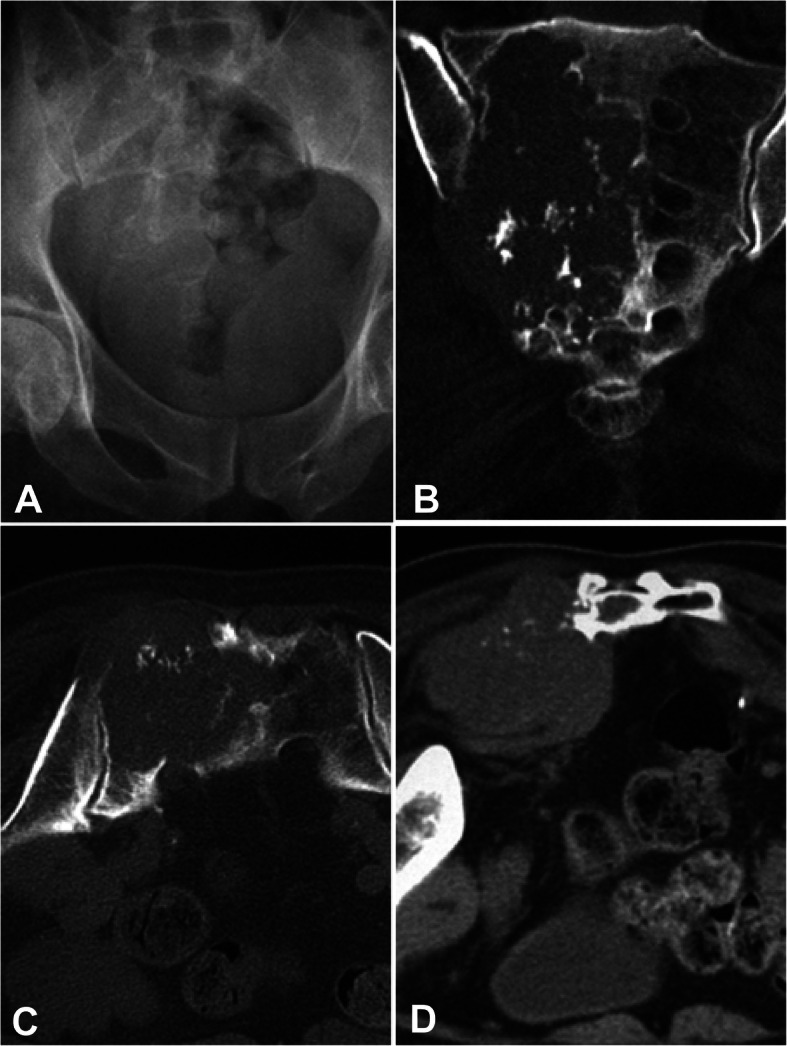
A 70-year-old man had right leg pain for 3 years and was diagnosed with sacral dedifferentiated chondrosarcoma. a Plain radiograph showed irregular bone destruction on the right side of sacrum. b-d Computed tomography revealed irregular bone destruction on the right side of sacrum with punctiform calcification and a soft tissue mass
